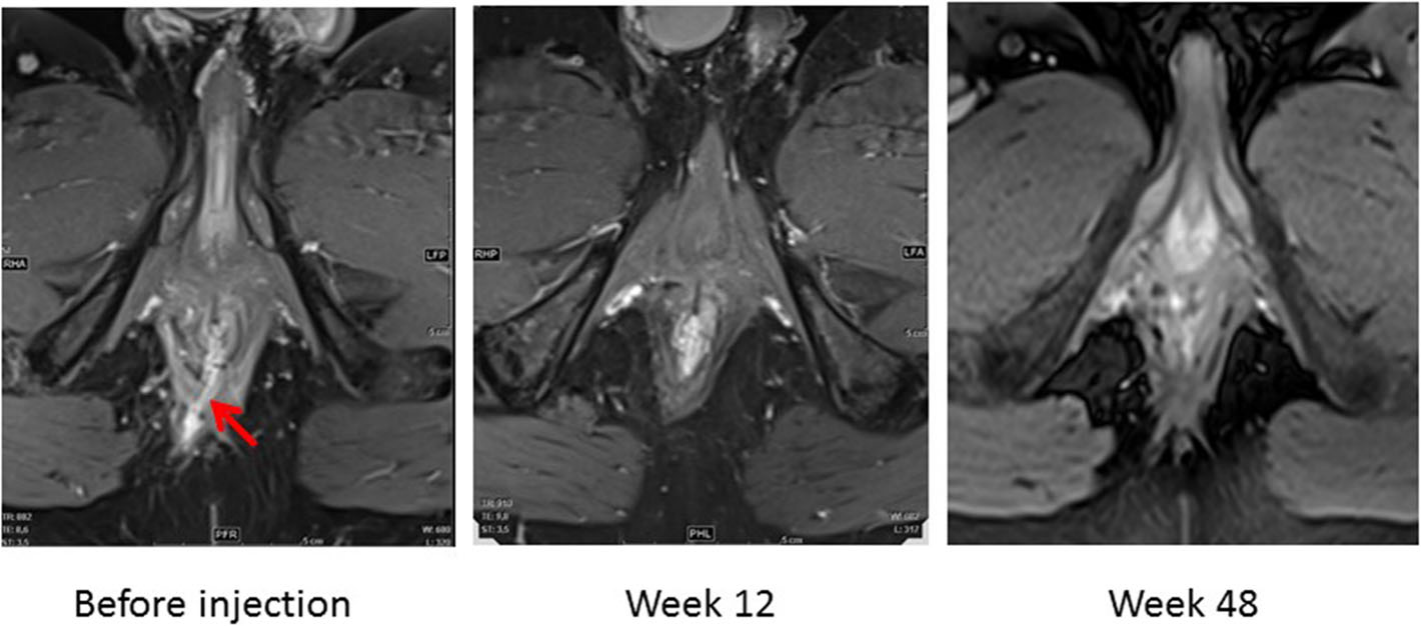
MRI images at baseline, 12 weeks, and 48 weeks. These perianal examinations of MRI results generated using T1 post-contrast (gadolinium) sequences show the disappearance of the fistula tract (red arrow) at week 12, which was confirmed at week 48, indicating complete healing of the fistula
